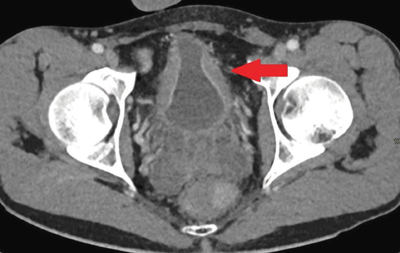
A 28-year-old patient. A contrast-enhanced abdominal CT scan showing the hypervascular mucosa and wall thickening of the middle part of the bladder with an ‘hourglass’ appearance (red arrow).
